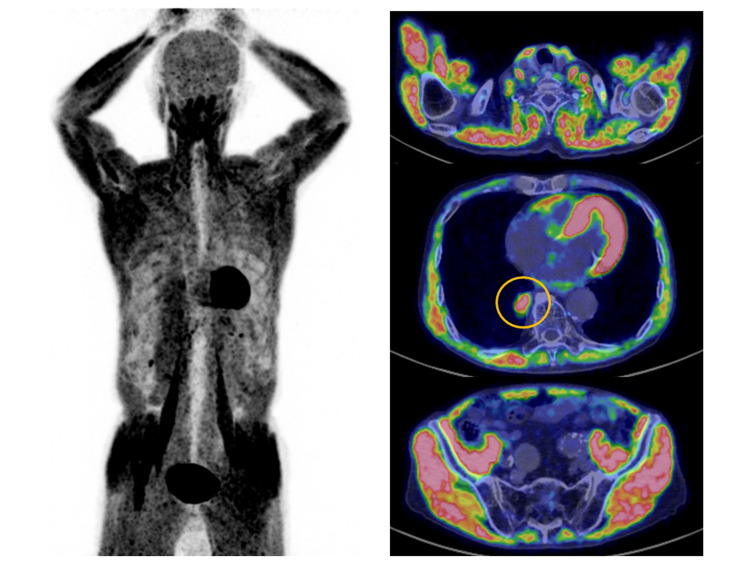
PET/CT image PET/CT: Positron emission tomography-computed tomography. High levels of 8F-fluorodeoxyglucose (FDG) accumulation were observed in skeletal muscle throughout the body. Accumulation was also observed in a lesion on the right lower lobe that was suspected to be a relapse of lung cancer (circle).
